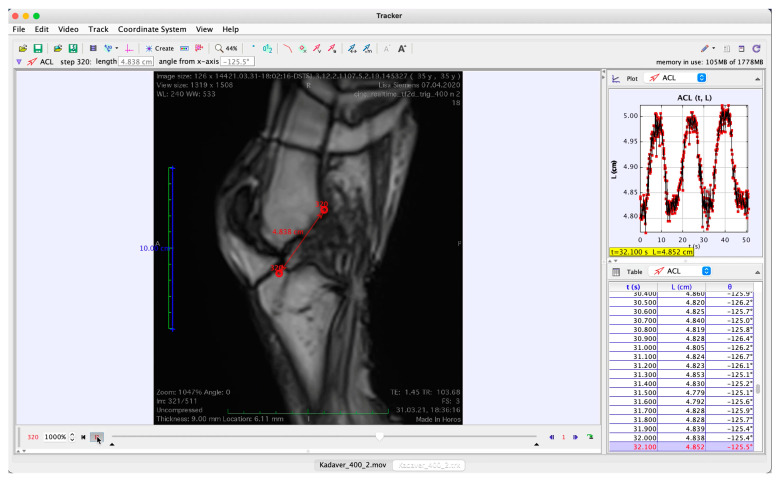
Screenshot of MRI cine sequence with points tracked for the estimation of ACL elongation. The red dots represent the points tracked during test execution.
